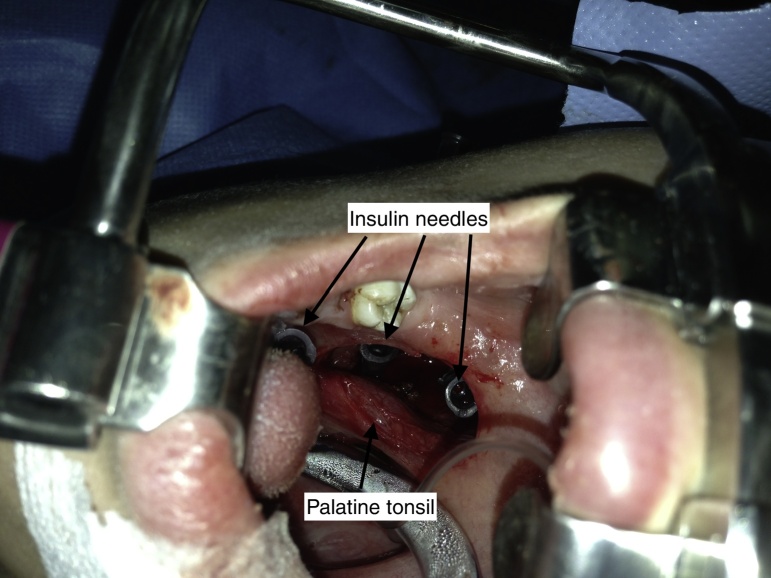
Intraoperative view: insulin syringe needles used as landmarks in intraoperative X-ray imaging and tissue dissection.
